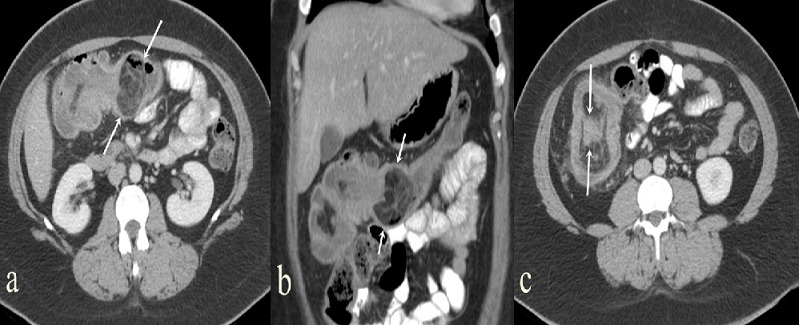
(A) in IV-Oral contrast abdominal computed tomography with axial section and coronal reformat image; (B) a mass lesion (arrows) is seen in the upper quadrant of the iliocecal junction with heterogeneous fat density and septal structures at this level. Axial view of IV-Oral contrast abdominal CT; (C) the herniation of the ileum into colonic pouch is seen (arrows)
